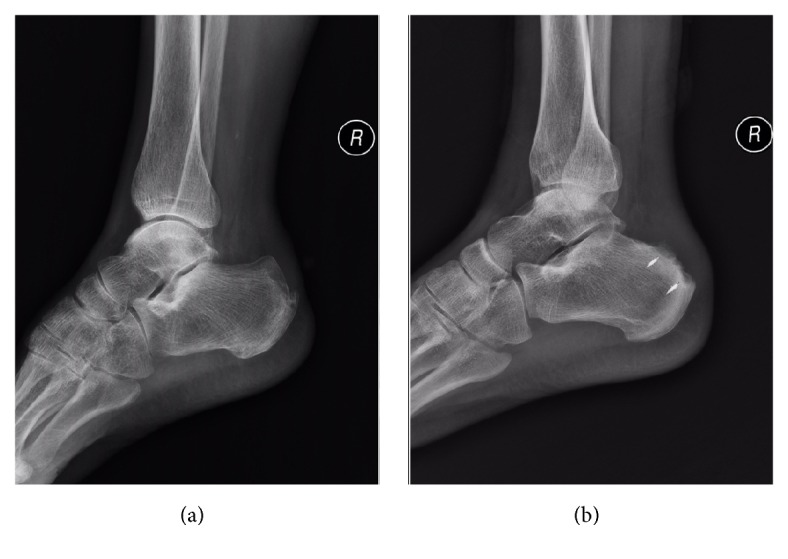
The standing lateral foot X-ray preoperatively and postoperatively showed complete excision of posterosuperior calcaneal prominence. (a) Preoperative X-ray showed obvious posterosuperior calcaneal prominence. (b) Postoperative X-ray showed the location of two suture anchors and the complete excision posterosuperior calcaneal prominence.
